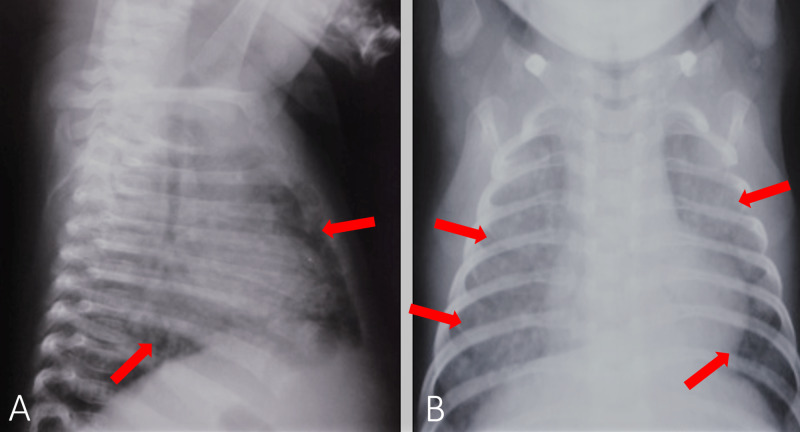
Chest radiograph. (A) Lateral view - diffuse opacifications of lung fields. (B) Anterior-posterior view - bilateral diffuse nodular infiltrate.
